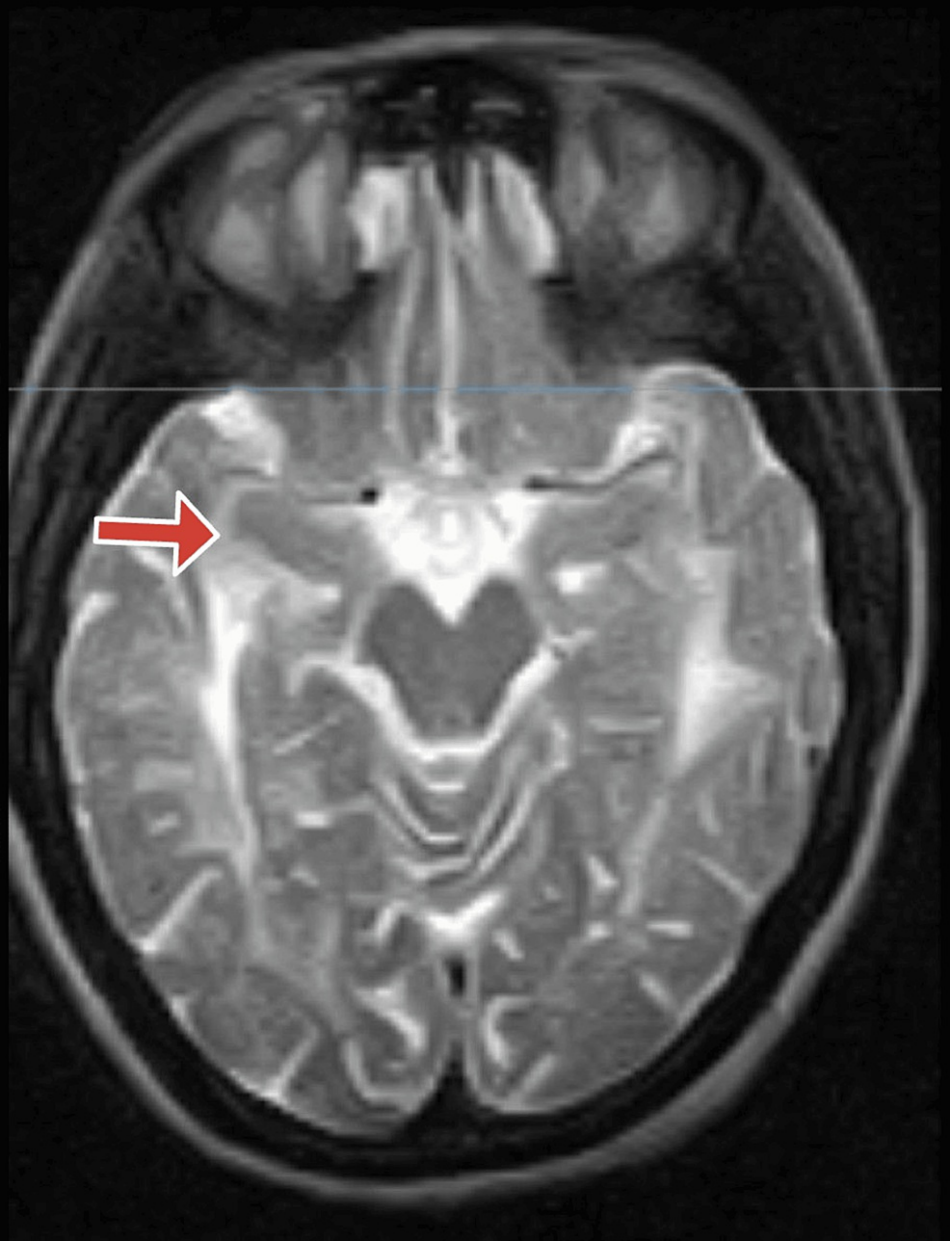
An MRI image of the brain with a red arrow showing white matter enhancement in the temporal lobe
